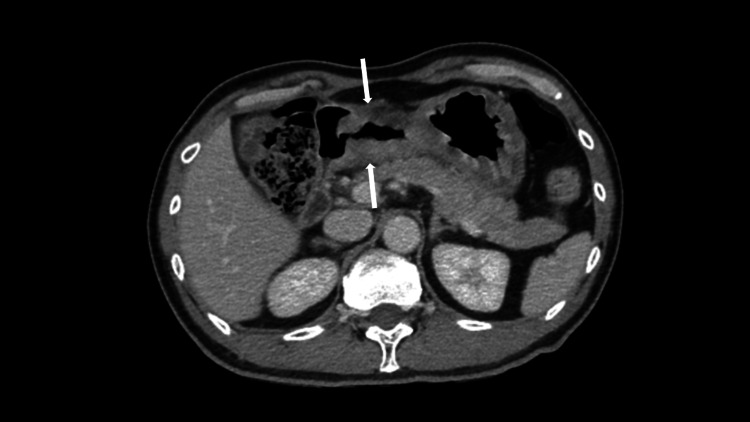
Contrast enhanced CT scan showing thickened gastric antrum anterior and posterior walls (arrows). CT: Computed tomography
